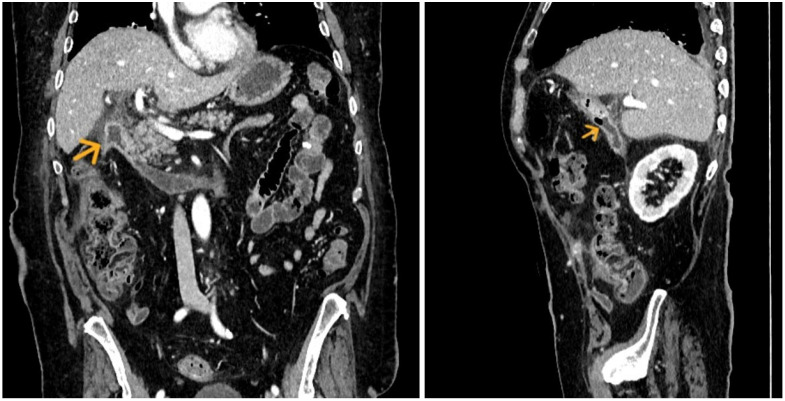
Abdominal CT scan with intravenous contrast uptake demonstrating duodenum recanalization after surgical drain’s removal. Yellow arrow: duodenal recanalization.
